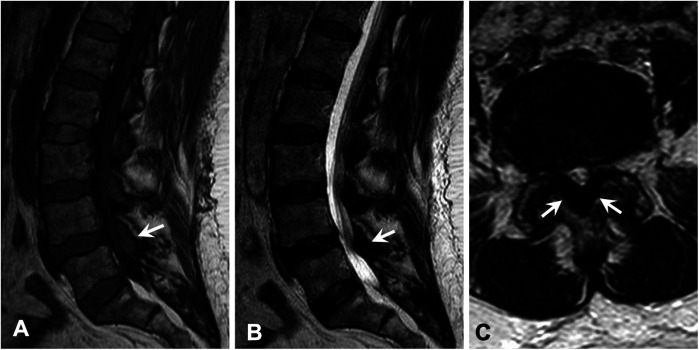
Magnetic resonance imaging of the lumbar spine demonstrated severe thickening of the ligamentum flavum at the level of L4–5 (arrow) with associated abnormal T1 hyperintensity, which caused severe narrowing of the spinal canal and bilateral neural foramina. Similar lesions were also seen at the levels of L1–4 to a lesser extent. (A) Sagittal T1-weighted image. (B) Sagittal T2-weighted image. (C) Axial T2-weighted image.
